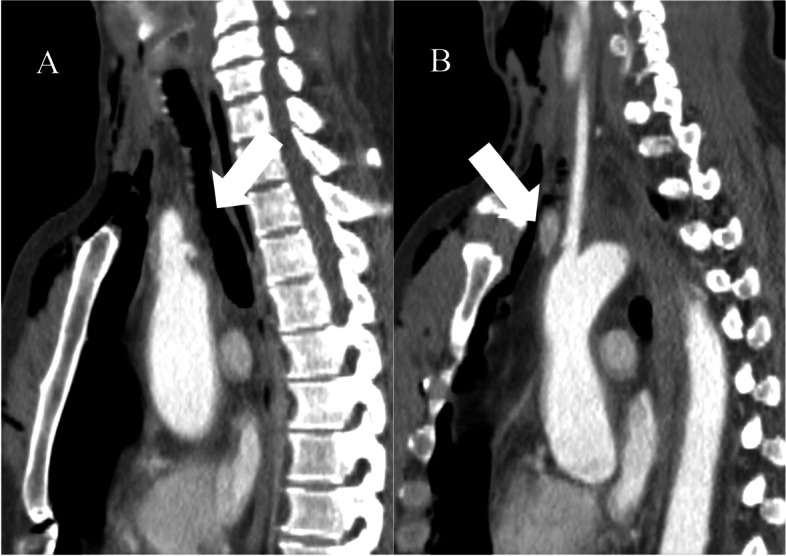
Contrast computed tomographic (CT) images during the emergency transportation. CT showed transections in the brachiocephalic artery (BCA) (A) and left carotid artery (LCA) (B)
